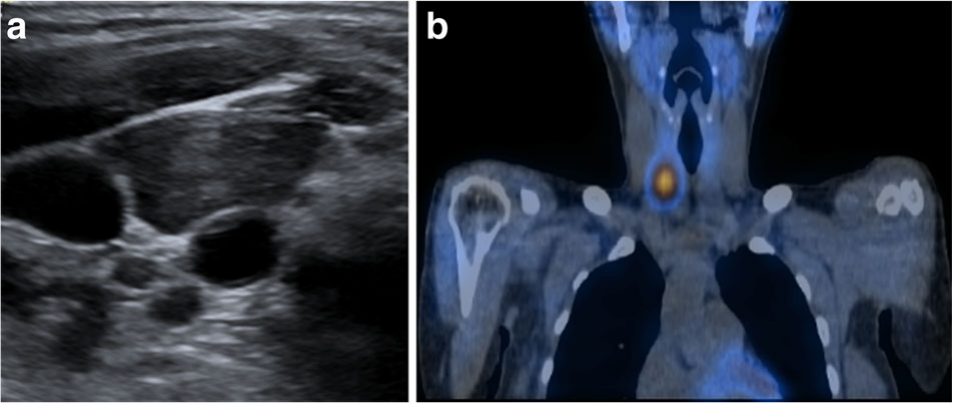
a, b Ultrasound and SPECT/CT showed a 15-mm right inferior parathyroid adenoma with residual high uptake
